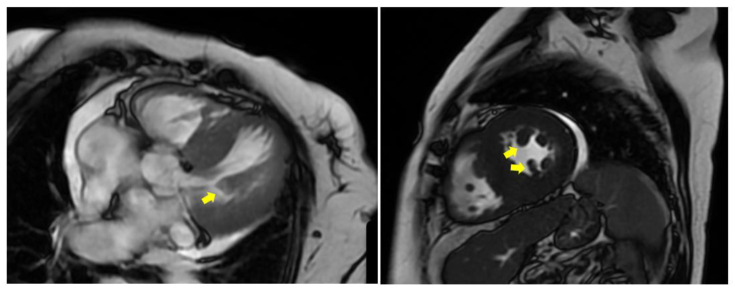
Cardiac MRI. PM hypertrophy in a patient with HOCM (yellow arrows). (MRI: magnetic resonance imaging, HOCM: hypertrophic obstructive cardiomyopathy, PM: papillary muscle).
